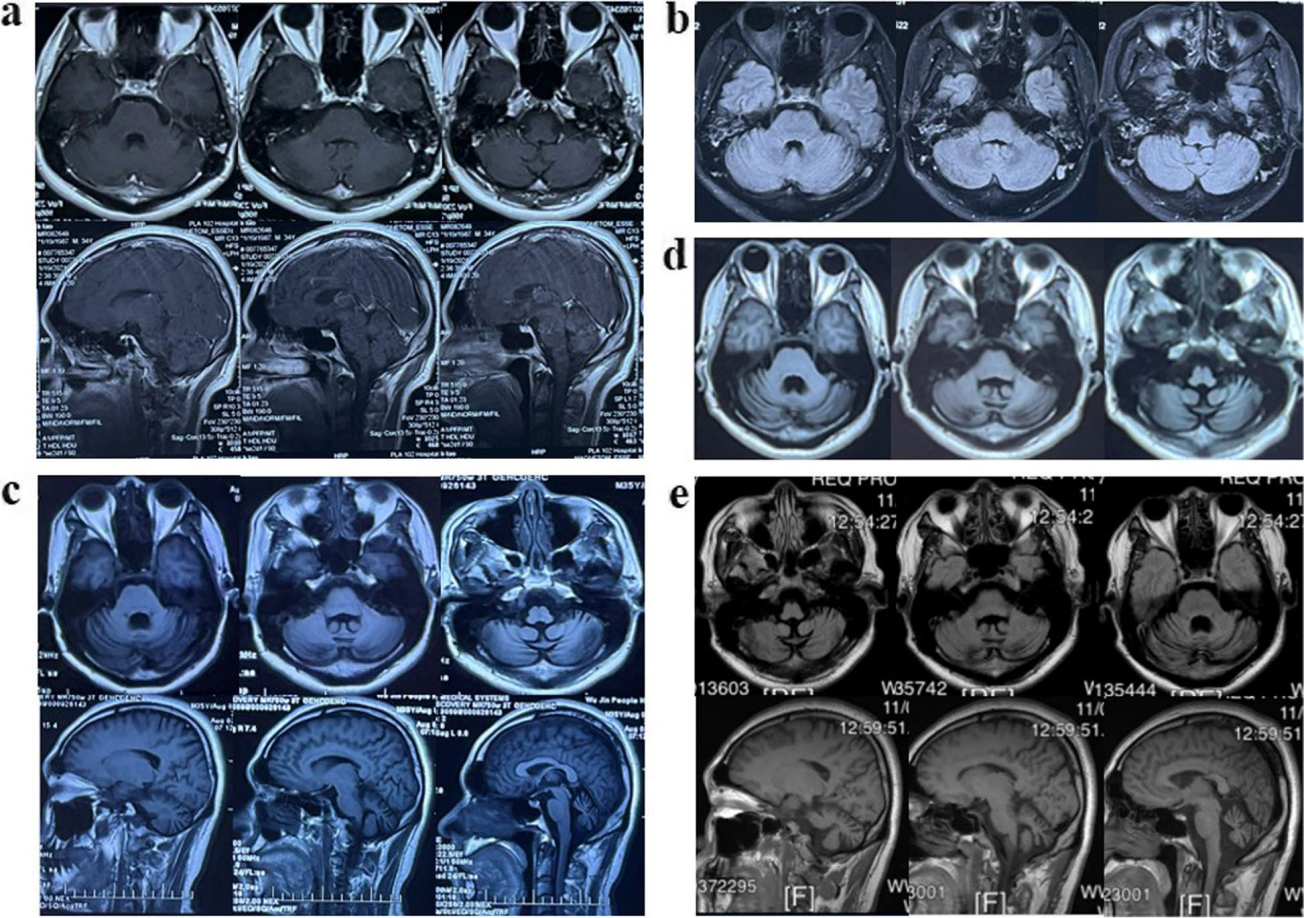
Panels a-e show head MRI scans from January 2021 (a), December 2021 (b), August 2022 (c), December 2023 (d), and November 2025 (e), respectively. These images show the continued progression of cerebellar atrophy without damage to other brain structures
